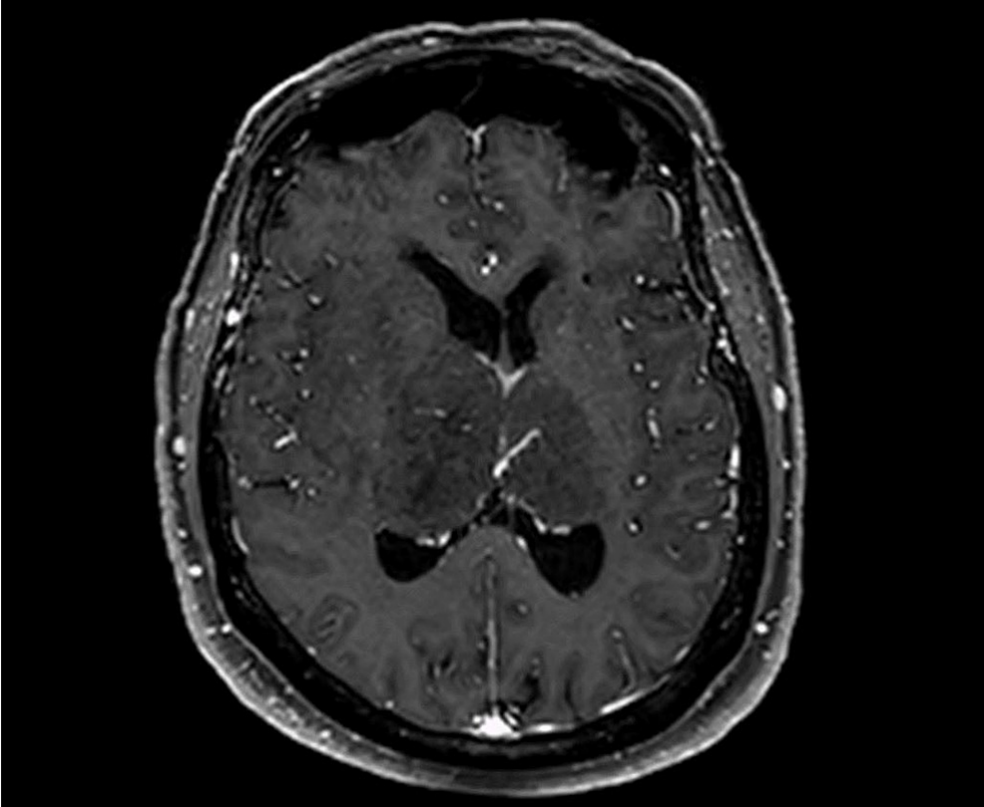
Axial T1-weighted post-contrast MRI The image demonstrates non-enhancing expansile lesions in the right greater than left thalami.
